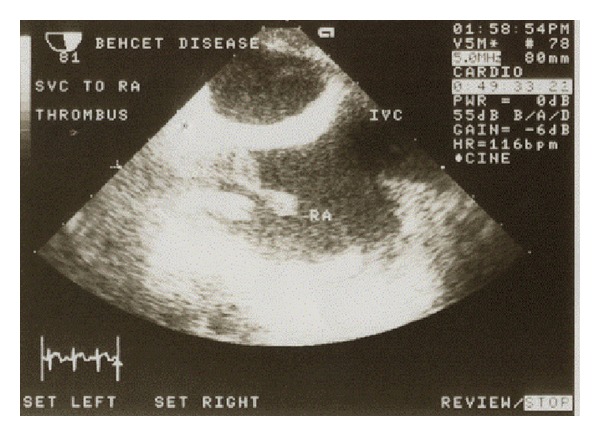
Transesophageal echocardiogram (Triplex) in patient A showing a sizeable (3 cm) pedunculated thrombus, which was oscillating in the right atrium (RA).
